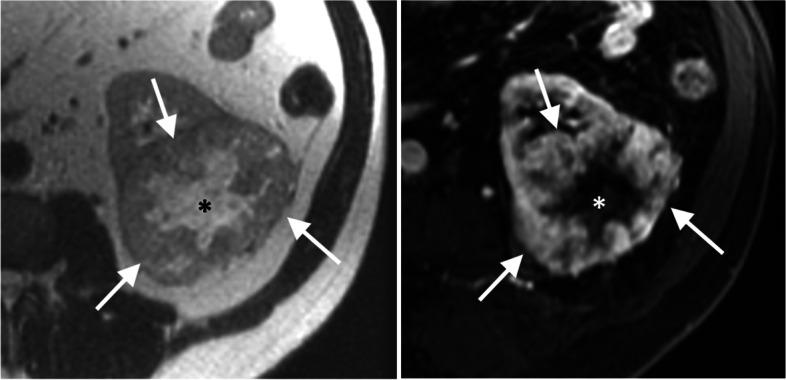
62-year old man with sarcomatoid RCC with no visible T2LIA. T2-weighted axial image (A) and post-contrast T1-weighted image (B) shows 8.5-cm avidly enhancing left kidney mass (arrows) with large central necrosis (*) but no measurable T2LIA. Baseline work-up revealed no metastatic disease. Radical nephrectomy was done after which pathological analysis showed 8.8-cm clear cell RCC with focal sarcomatoid differentiation and muscular venous branch invasion. After adjuvant treatment with pazopanib, patient developed left lower lobe lung metastasis 670 days after diagnosis and was subsequently treated with multiple lines of systemic treatment including lenvatinib, pembrolizumab, and nivolumab. Patient is still alive after 3397 days
